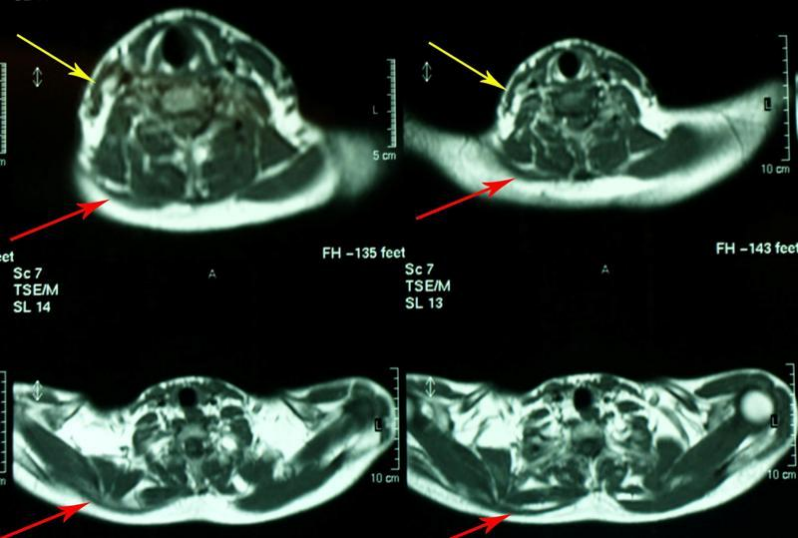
Magnetic resonance imaging of the neck showing muscle wasting of the right trapezius (red arrows) and sternocleidomastoid (yellow arrows) muscles.
